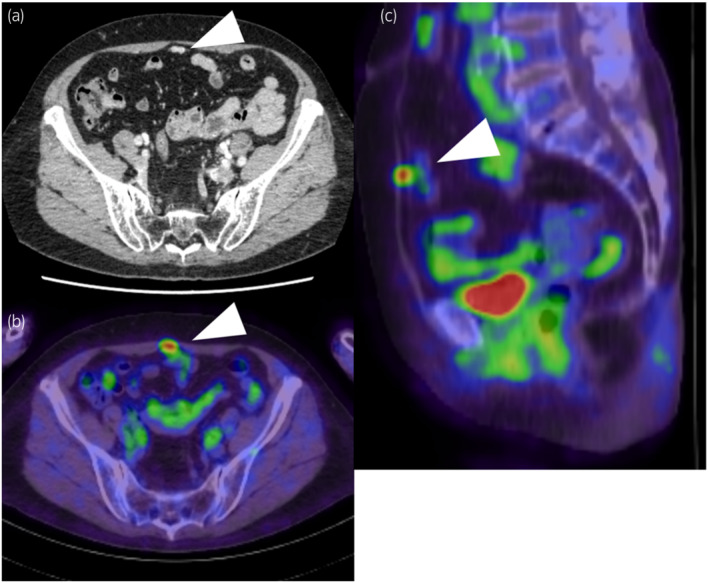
Images of the lower abdominal tumor (arrowhead). (a) A 1.2‐cm tumor was found in the midline of the lower abdominal wall on an axial view of the computed tomography (CT). (b), (c) Axial and sagittal view of fluorodeoxyglucose positron emission tomography‐computed tomography (FDG‐PET/CT) showed intense FDG uptake (maximum standardized uptake value, SUV_max_, 4.51).
